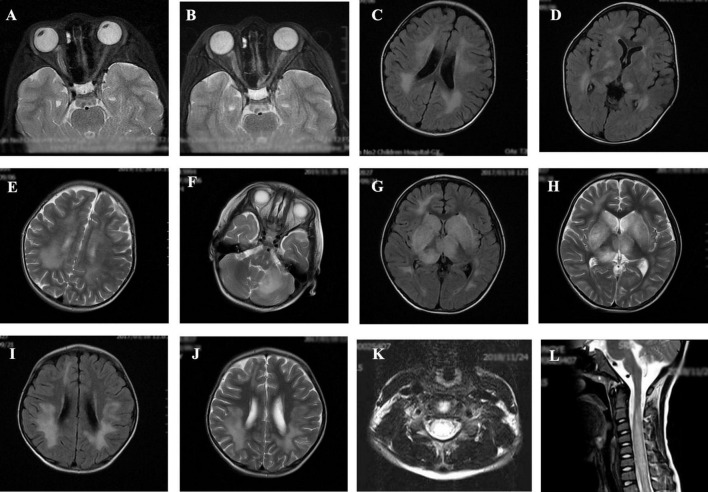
Magnetic resonance imaging (MRI) results of acute disseminated encephalomyelitis (ADEM) children with or without myelin oligodendrocyte glycoprotein-immunoglobulin G (MOG-IgG. (A,B) (T2-axial) cerebral MRI of a 7-year-old boy with MOG-IgG seropositive ADEM showed small and blurred lesions of optical nerve. (C–F) [T2-axial, fluid-attenuated inversion recovery (FLAIR)-axial] cerebral MRI of a 2-year-old girl with MOG-IgG showed large, blurred lesions in bilateral white matter areas of frontal, parietal, and temporal lobes, bilateral basal ganglia, bilateral thalamus, and left cerebellar hemisphere. (G,H) (T2-axial, FLAIR-axial) cerebral MRI of a 4-year-old boy without MOG-IgG revealed a lesion in bilateral basal ganglia, thalamus, periventricular white matter, and right frontal lobe. (I,J) (T2-axial, FLAIR-axial) cerebral MRI of a 4-year-old girl with MOG-IgG seronegative ADEM showed large and blurred lesions in bilateral white matter areas of the frontal parietal. (K,L) (Sagittal-T2) Spinal MRI of a 2-year-old girl with MOG-IgG revealed prominent involvement of cervical and thoracic spinal lesion (≥3 segments).
